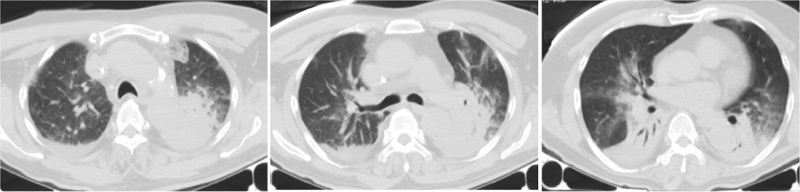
Chest computed tomography of Case 3, a 58-year-old male, showing patchy consolidation of both lungs with blurred edges, and a small right pleural effusion.
